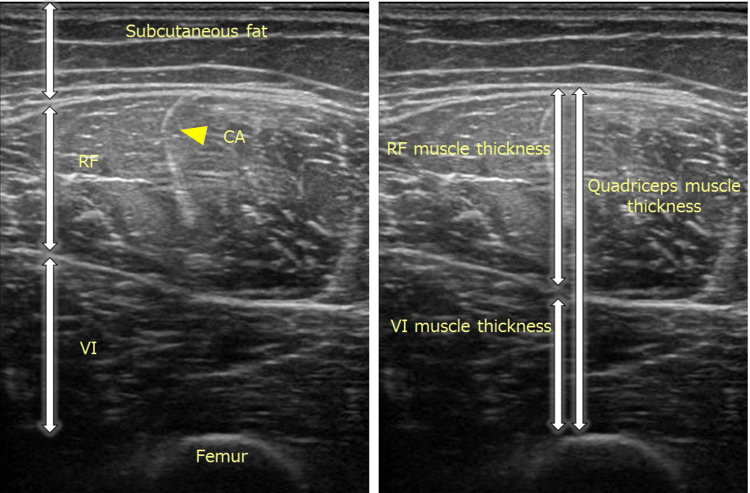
Ultrasound images showing the method for measuring the muscle thickness of the quadriceps femoris. The right panel shows the lateral view, while the left panel presents the medial view. Abbreviations: RF, rectus femoris; VI, vastus intermedius; CA, central aponeurosis
